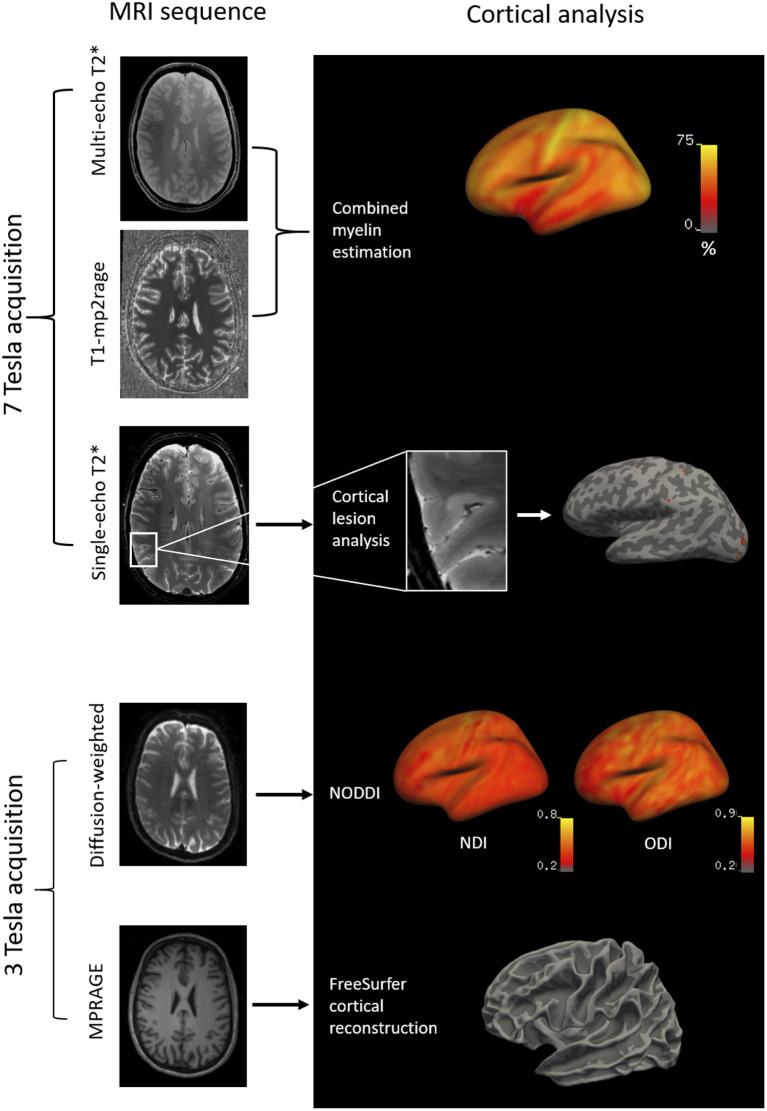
Methodology of the imaging analysis: 7T multi-echo T_1_-MP2RAGE and T${}_{2}^{*}$ sequences were combined by independent component analysis to obtain cortical CME maps. Cortical lesions were segmented on 7T single-echo T${}_{2}^{*}$ sequences and projected to the surface of the subject. Diffusion-weighted images at 3T (b-values=1,000 and 5,000 s/mm^2^; diffusion directions=64 and 128) were acquired to obtain cortical NODDI maps. T1-weighted MPRAGE images at 3T were acquired for FreeSurfer cortical reconstructions. MP2RAGE, dual magnetization-prepared rapid gradient echo; MPRAGE, magnetization prepared rapid acquisition gradient echo.
